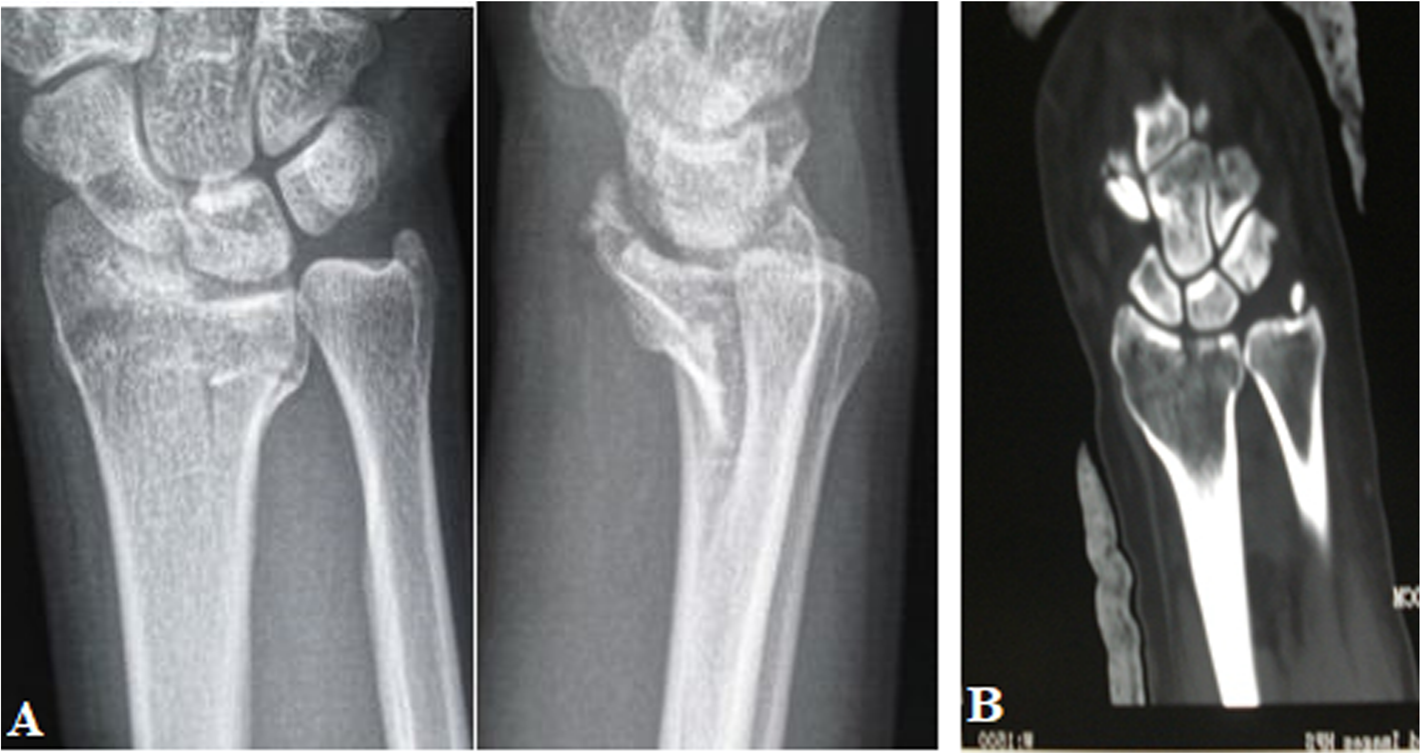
PA X-ray (a) and CT scan (b) of the collapse single-column DRDPF
